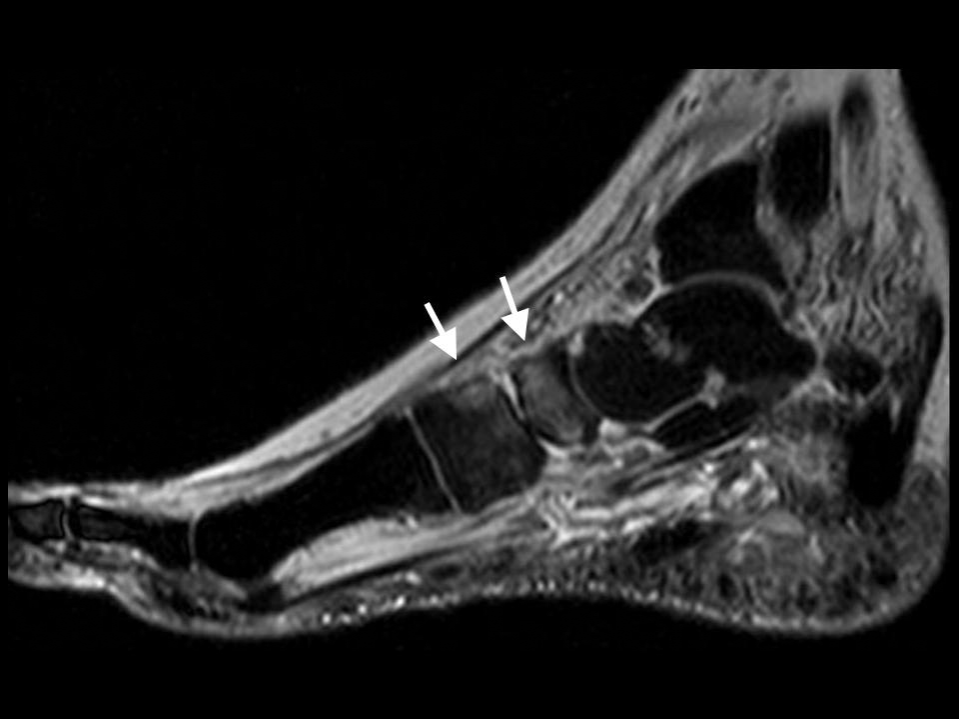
Same foot as in Figure 7. MRI sagittal STIR-sequence shows regression of bone marrow edema (white arrows) and soft tissue edema.
